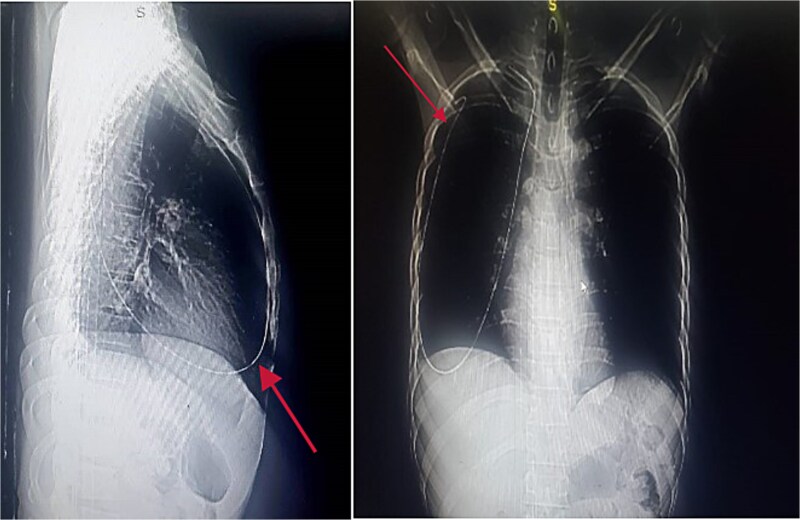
Chest X-ray posterior anterior and lateral view showing the intrathoracic metallic guide. The wire’s thin, linear shape is clearly visible, with an abrupt change in course around the cupola indicating its unintended migration from the subclavian vein into the chest cavity.
